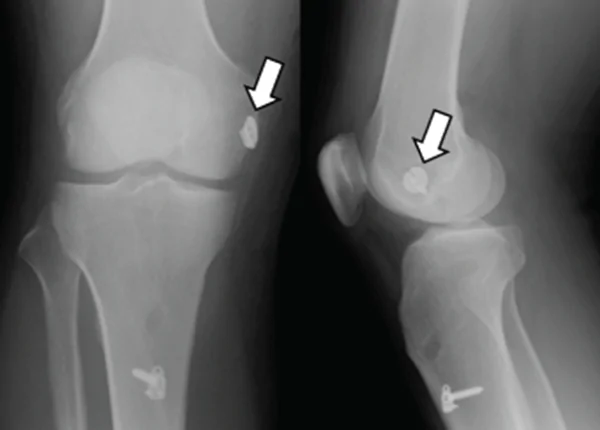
A radiograph of the right knee after revision surgery demonstrating that the repositioned EndoButton was augmented by TightRope ABS button (white arrow).
